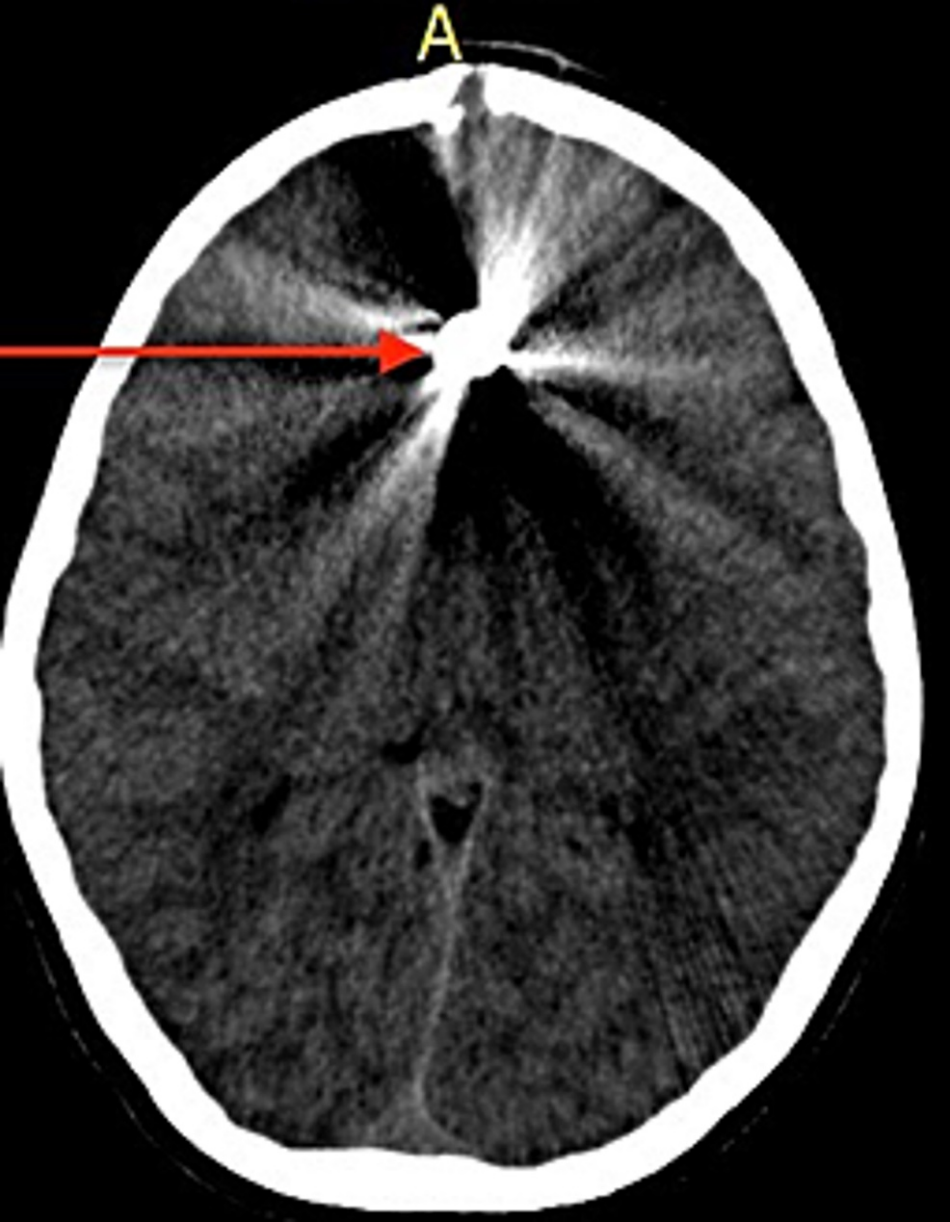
Computed tomography scan of the head showing an axial view in which a metallic foreign body is seen with a beam hardening artefact
